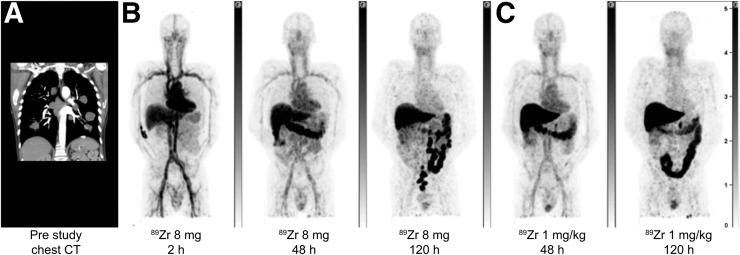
Maximum-intensity projections of patient 2: prestudy CT scan showing multiple lung lesions (A), ^89^Zr-GSK2849330 PET after 8 mg total mAb dose at 2, 48, and 120 h after infusion (B), ^89^Zr-GSK2849330 PET after 24 mg total mAb dose at 48 and 120 h after infusion (C).
